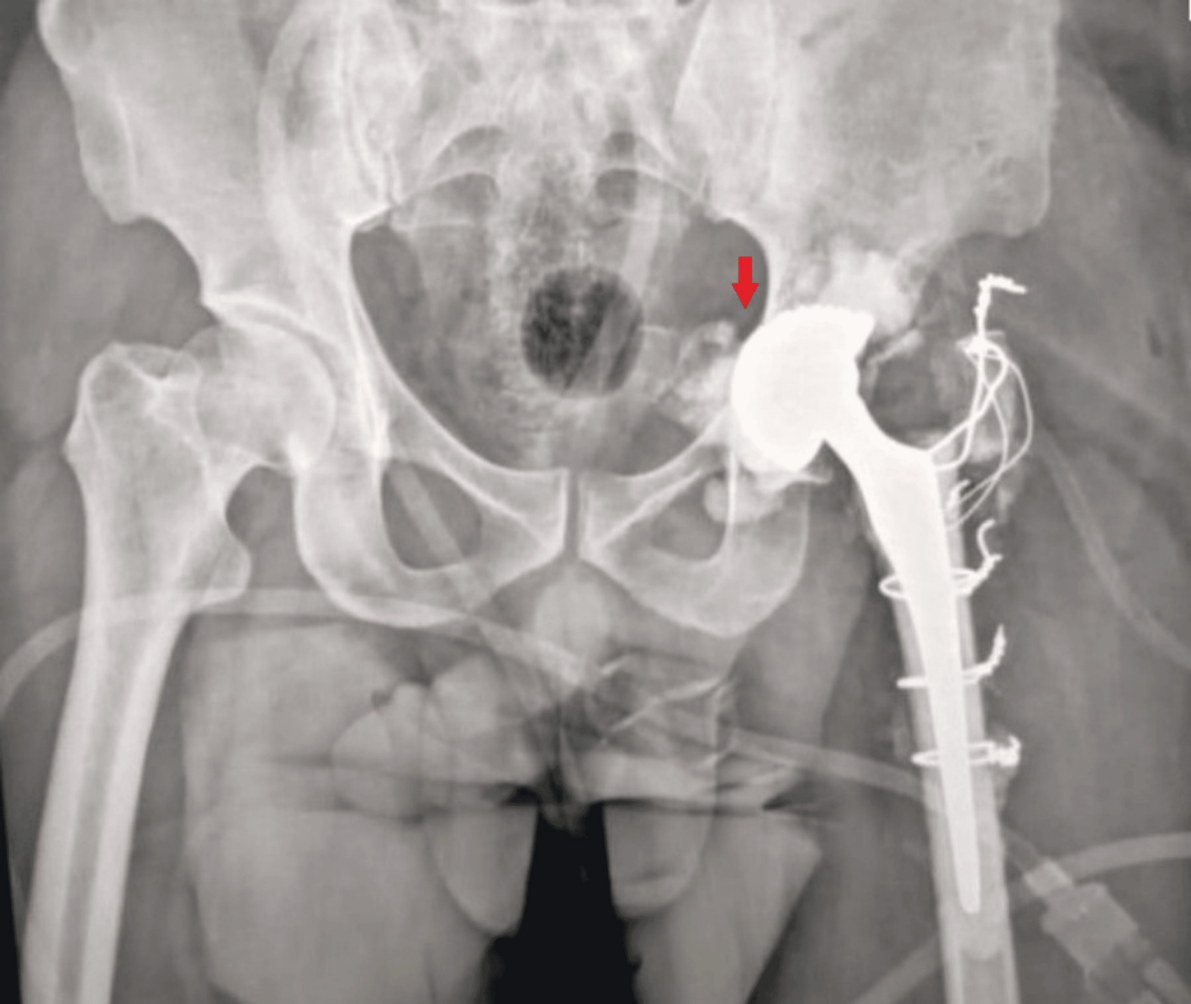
Radiograph following revision surgery with dual mobility total hip arthroplasty (red arrow), of the same patient in Figure 4 and Figure 5
